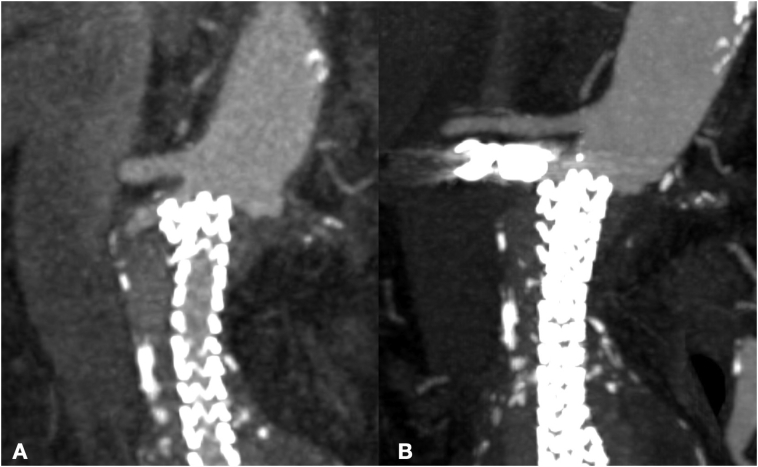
Preoperative CT Angiography with endoleak (A) and 6-months postoperative CT Angiography (B).
